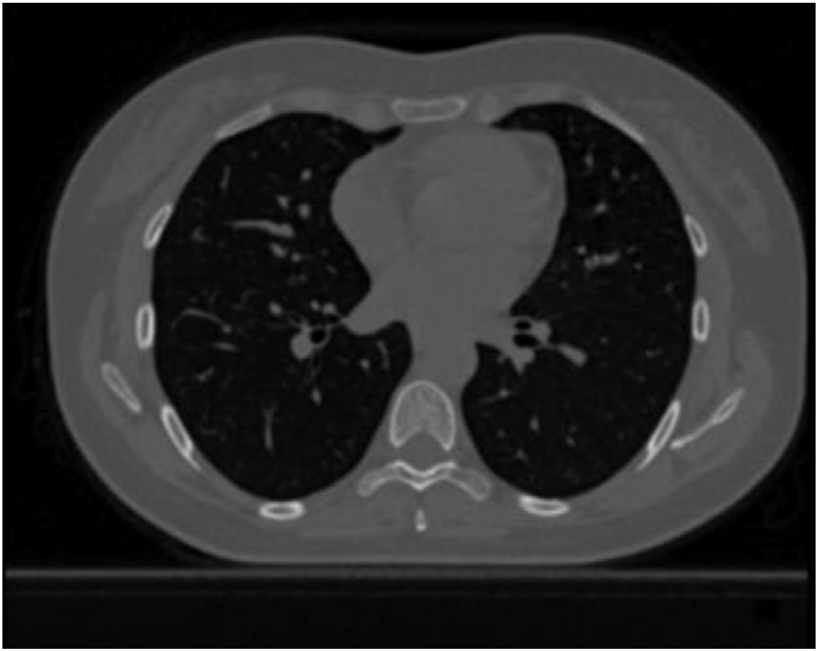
Chest CT slice of patient (A2) with no abnormalities.
